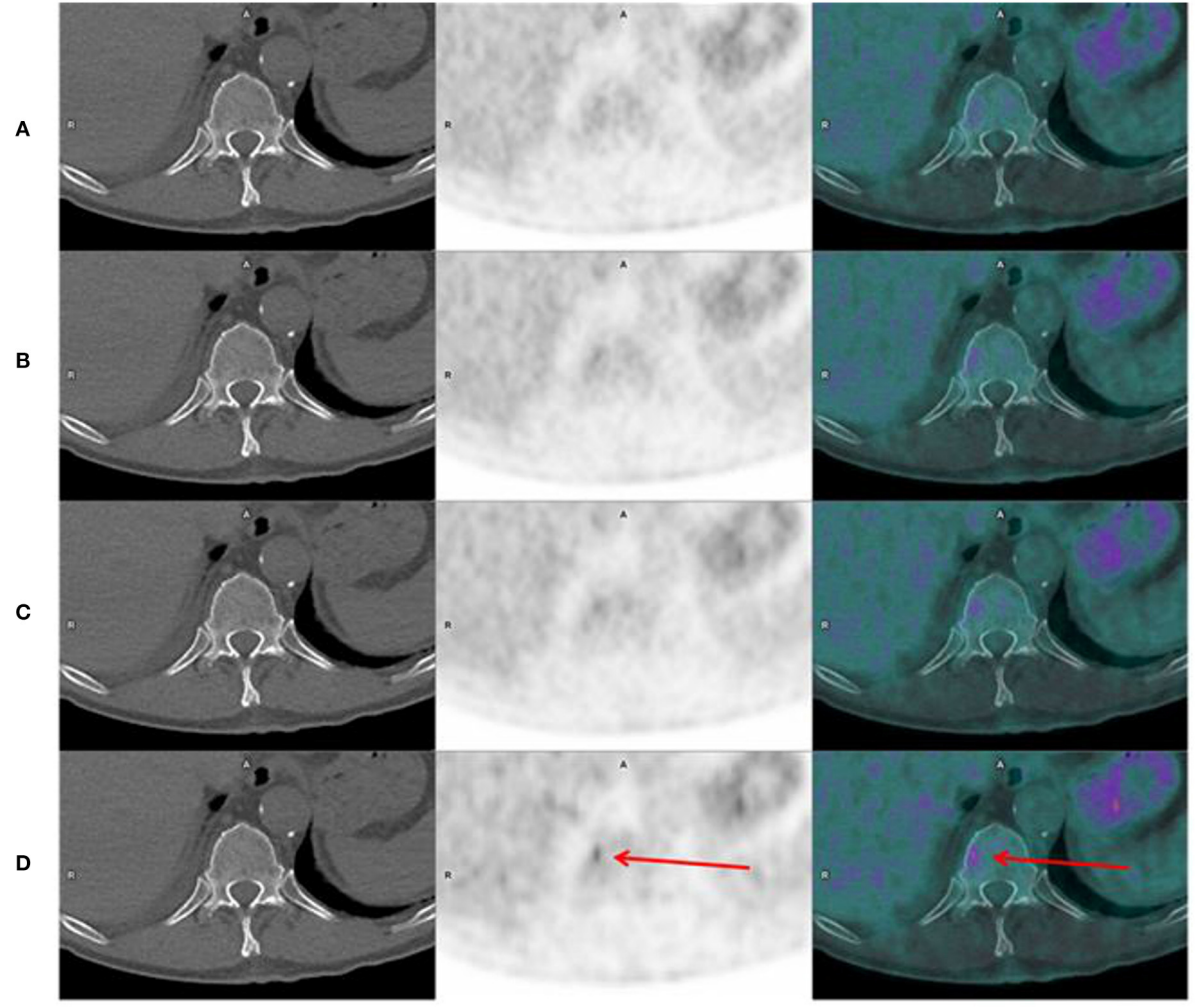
Axial CT, PET, and fusion images in a 65 years old man, showing a bone focal uptake in T11, on the “Analog-like” acquisition, which disappears when increasing acquisition time, and corresponding actually to noise [(A-D) from top to bottom, respectively, 120, 90, 60, and 30 s per bed position].
